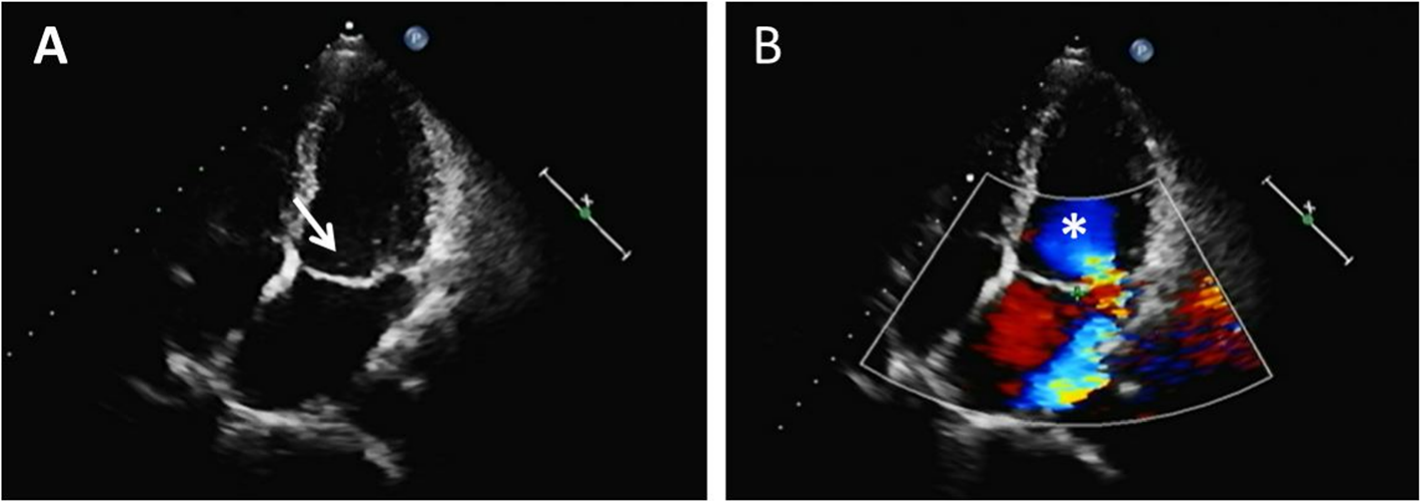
Echocardiography shows moderate mitral insufficiency in a 32-year-old TA patient with elevated ASO. Two - dimensional echocardiography suggested mitral valve calcification and prolapse (a), and Color Doppler ultrasound detected a large number of regurgitation signals in left atrium (b)
